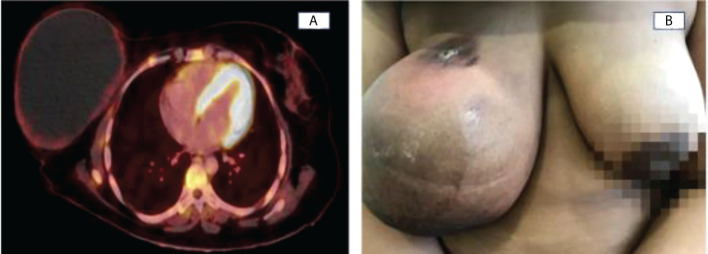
(A) Complete metabolic response due to persistence of a voluminous cystic left breast lesion with a thin wall of low uptake of FDG ((SUVmax:2,21) and complete disappearance of ipsilateral locoregional lymphadenopathy. (B) After completing NAC, the nodule at the union of the upper quadrants disappeared.
